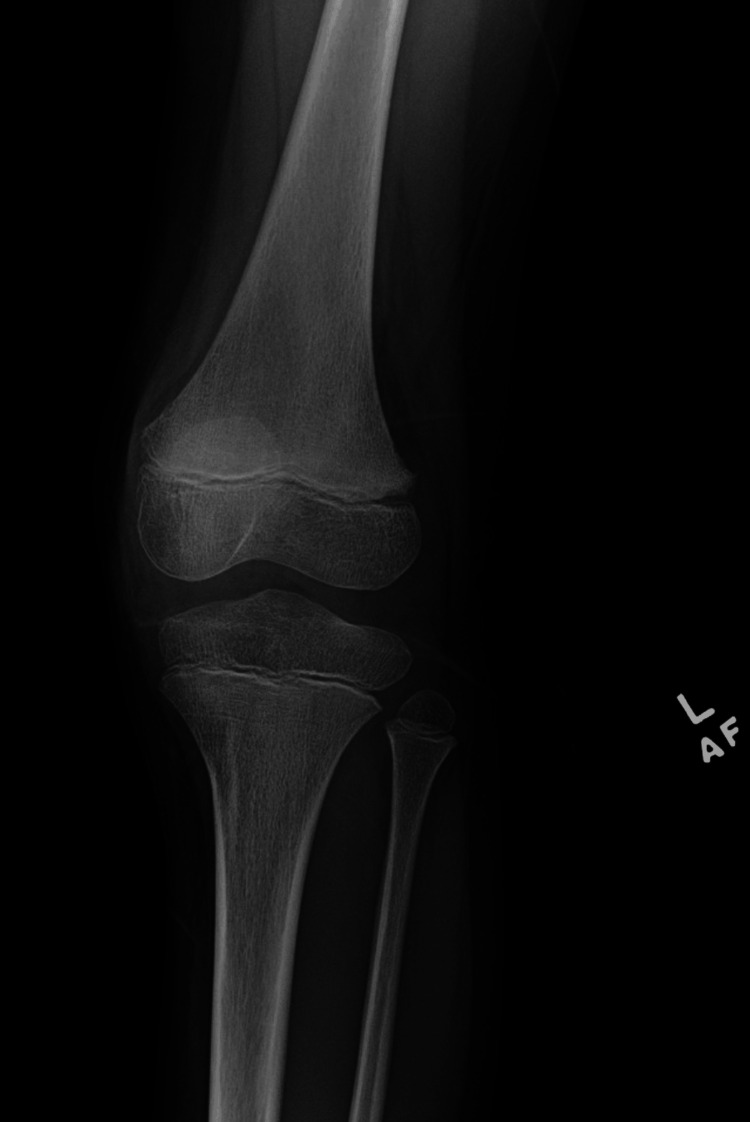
X-ray of the left knee showing soft tissue swelling of the medial knee
